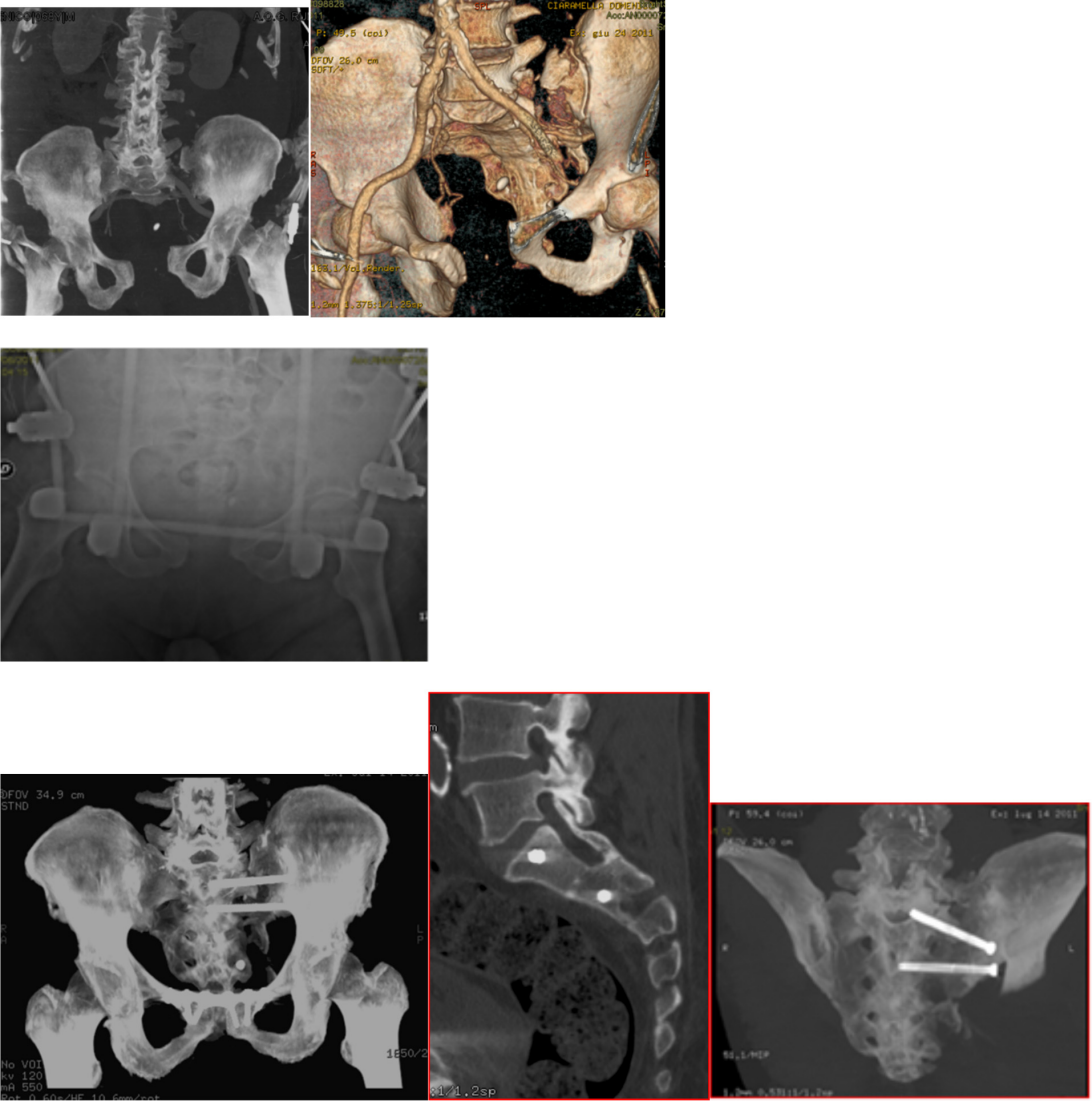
Case of a 55-year-old male patient who sustained a Type III Dennis fracture of the left S-I joint associated with pubic symphysis diastasis (type B1) following a fall from height (top pictures: coronal CT view and CT reconstruction). The injury was treated within few hours from the fall with external fixator (middle pic: AP plain radiograph of the pelvis) and he was then admitted to the ITU ward. This was followed by reduction and fixation with plate and screws 7 days after ITU admission (bottom-left picture). The treatment was completed 3 days after plating with S-I joint reduction and fixation with two percutaneous CT-guided screws within S1 and S2 vertebral body (last two bottom pictures).
